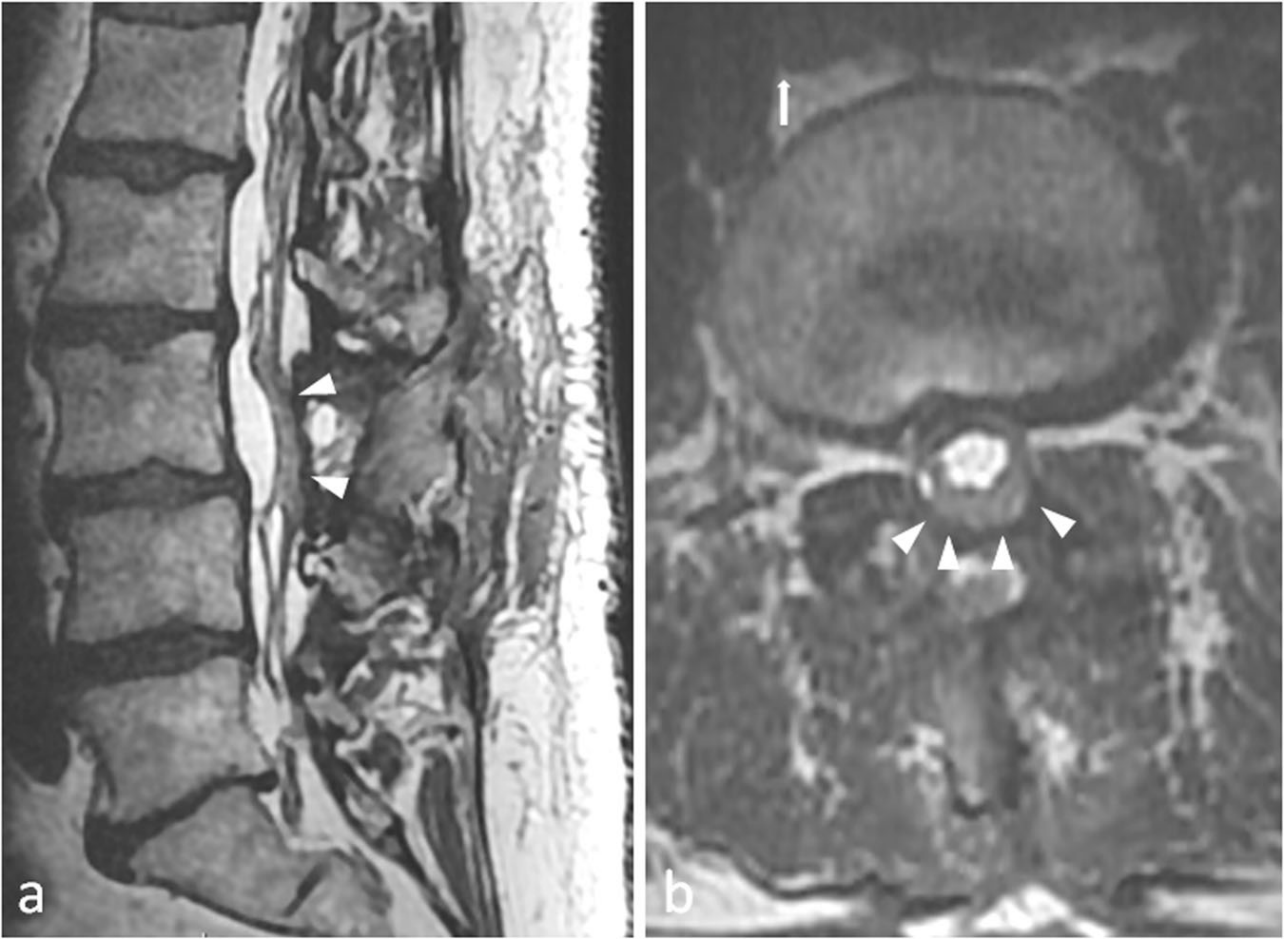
This patient was operated of a L3–L4 lumbar spine stenosis by L3 laminectomy. A dural tear occurred during surgery, and the dural sac was stitched. Although his former symptoms regressed, he reported non-systematised pain in the lower limbs that he did not experience before. MRI shows clumped nerve roots, with no fluid between the roots and the dural sac (arrowheads) which is suggestive of adherences in an arachnoiditis. Sagittal (a) and axial (b) T2-weighted images
